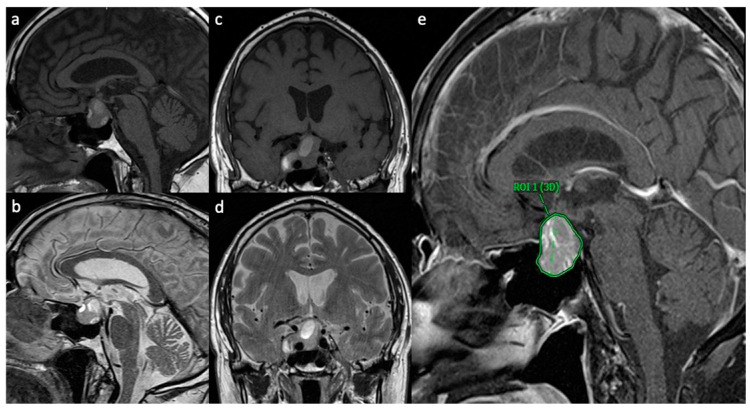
Representative MRI scans of H-PitNet with multiple hemorrhagic lesions. (a) Sagittal T1W MRI showing T1 hyperintense hemorrhagic lesions in a PitNet; (b) sagittal MRI showing T2 hyperintense lesions in a PitNet. The same lesions are displayed in the coronal view in (c) (T1W) und (d) (T2W). A sagittal view of contrast-enhanced T1W MRI is displayed in (e), showing an inhomogeneous contrast enhancement on the PitNet, which is marked as a 3D region of interest (ROI) for volumetry.
